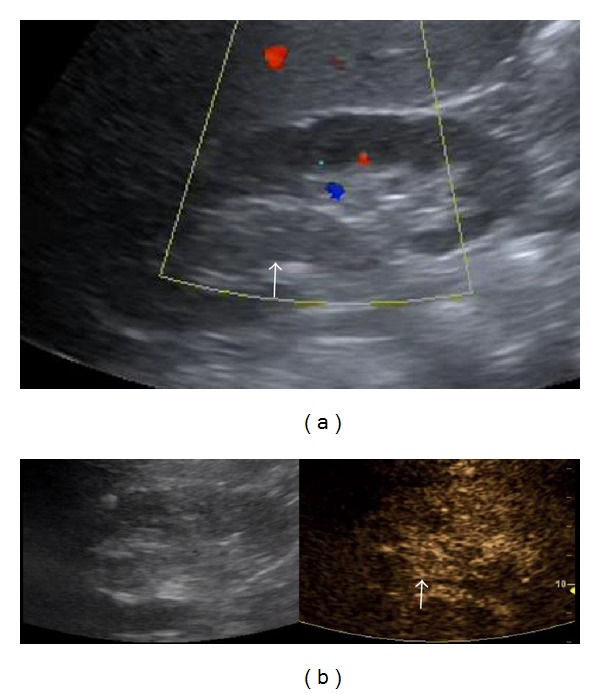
Transitional cell carcinoma: echogenic content is located in the upper part of the dilated pelvicalyceal system. It does not show blood flow on Colour Doppler US (arrow in (a)). However, it enhances on CEUS (arrow in (b)), due to its neoplastic nature.
